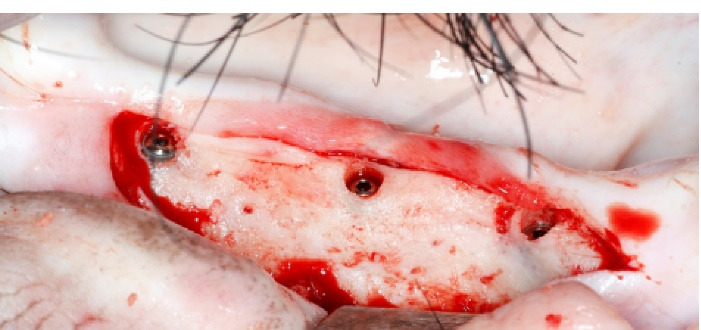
The implant axis was checked at each step. For each implant, a minimum torque of 35 N.cm was achieved, using a cover screw. Finally, the mucosa was stitched with FLEXOCRIN 4/0 (copolyamide) (B. Braun, Germany). Implant placement was checked on retro alveolar X-rays, with an alignment key.
